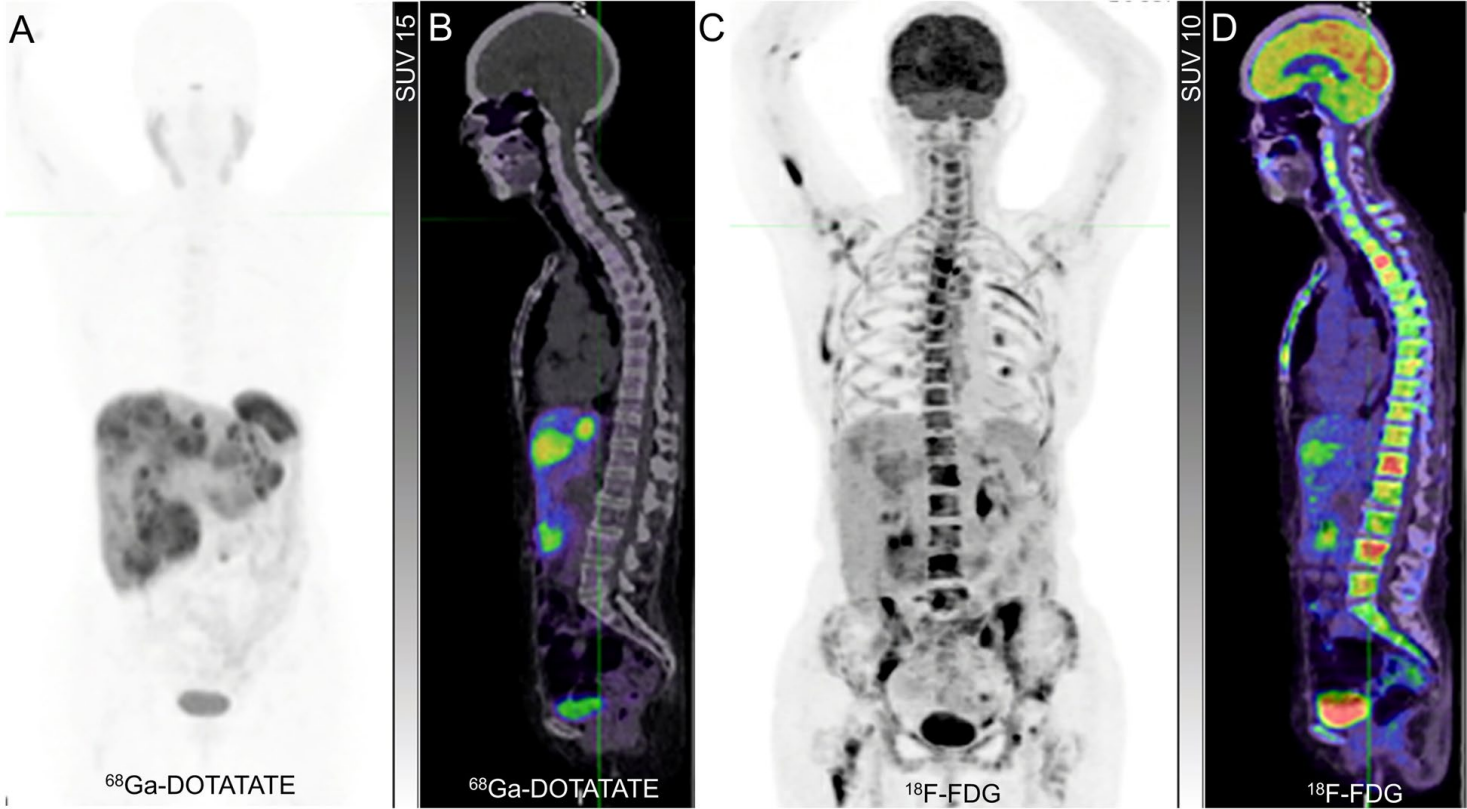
Discordant ^68^Ga-DOTATATE and ^18^F-FDG PET due to a second malignancy. A patient with a small intestinal NET, initially of G1 with Ki-67 2% but subsequently converted to G3 with Ki-67 30% based on later liver biopsy, with contemporaneous ^68^Ga-DOTATATE PET (A) and ^18^F-FDG PET (C) demonstrating concordant tracer avid known liver disease but extensive and heterogeneous ^18^F-FDG-avid/non-^68^Ga-DOTATATE avid in the axial and proximal appendicular skeleton, also demonstrated on sagittal fused PET/CT (B and D). Given the absence of skeletal uptake on the ^68^Ga-DOTATATE PET and high ^18^F-FDG uptake, bone marrow biopsy was performed demonstrating excess myeloid blasts consistent with early acute myeloid leukemia. Interestingly, blood counts were not significantly deranged at that time. No evidence of NET metastasis was identified on the bone marrow trephine
